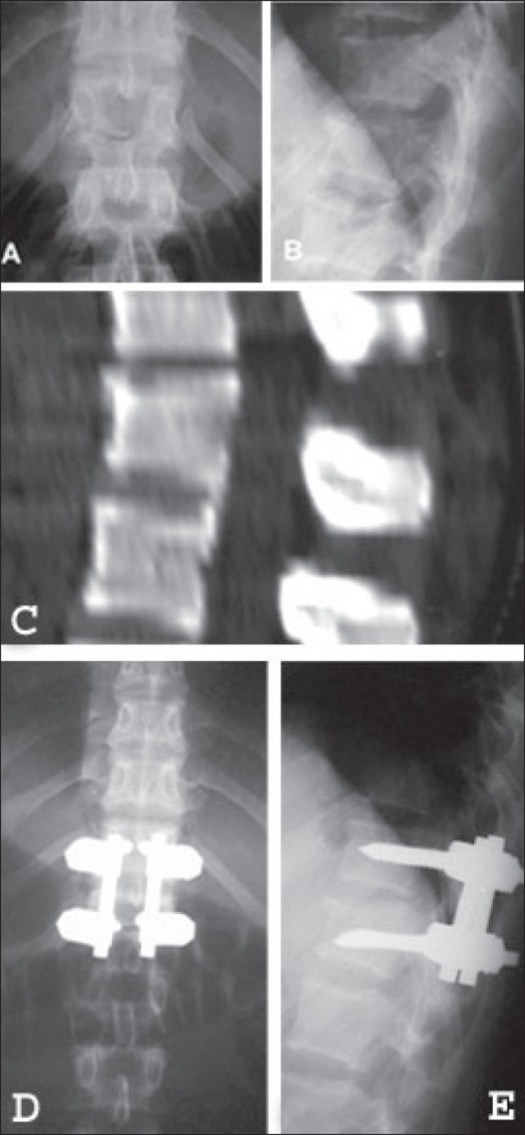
Preoperative X rays of dorsolumbar spine AP and lateral (A & B) shows a type B thoracolumbar fracture. Preoperative CT sagital reconstruction (C) shows the thoracolumbar fracture. The 2 years postoperative x-rays (D & E) show a posterior monosegmental fixation without complications
